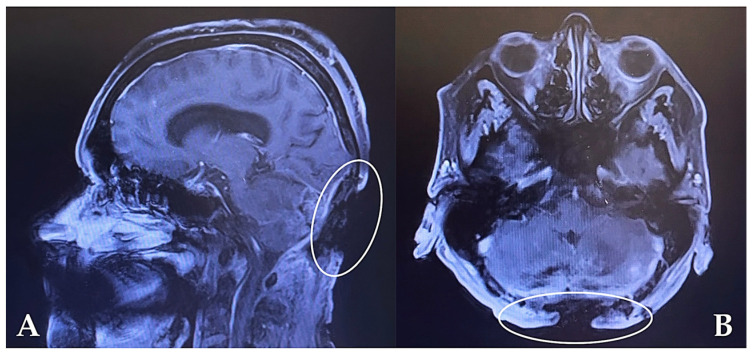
Contrast-enhanced MRI of the occipital region. (A) Sagittal view showing the occipital bone defect with dural involvement. (B) Axial view demonstrating the extent of the defect. Image courtesy of Dr. Iones Afana, used with permission.
